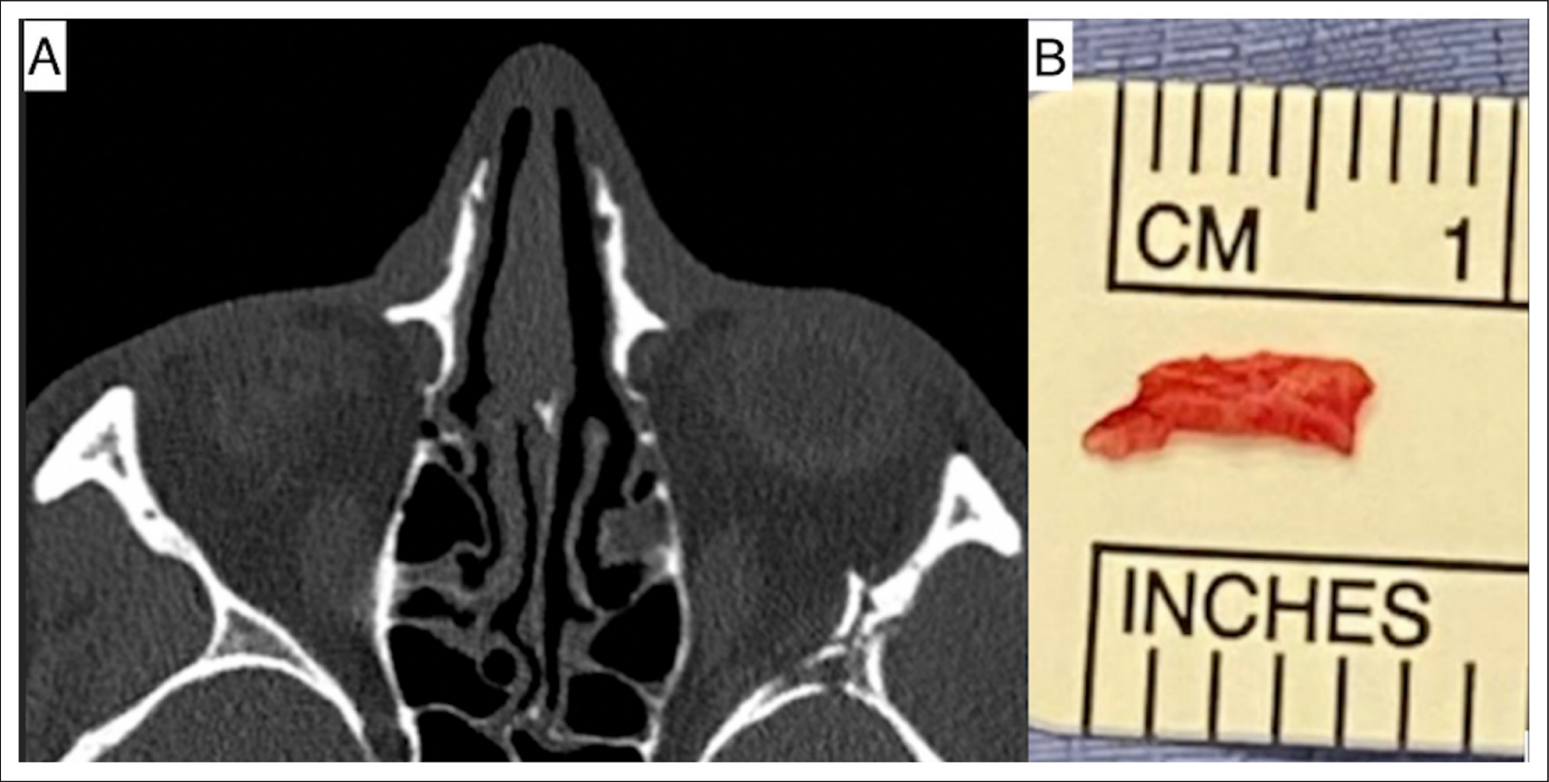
(A) Nonenhanced brain CT scan at admission. Bone view showing fracture of the lateral wall of the left orbit (white arrow), with a small area of haemorrhage in the left anterior temporal fossa. (B) Bony spur that was removed from lateral rectus muscle.
